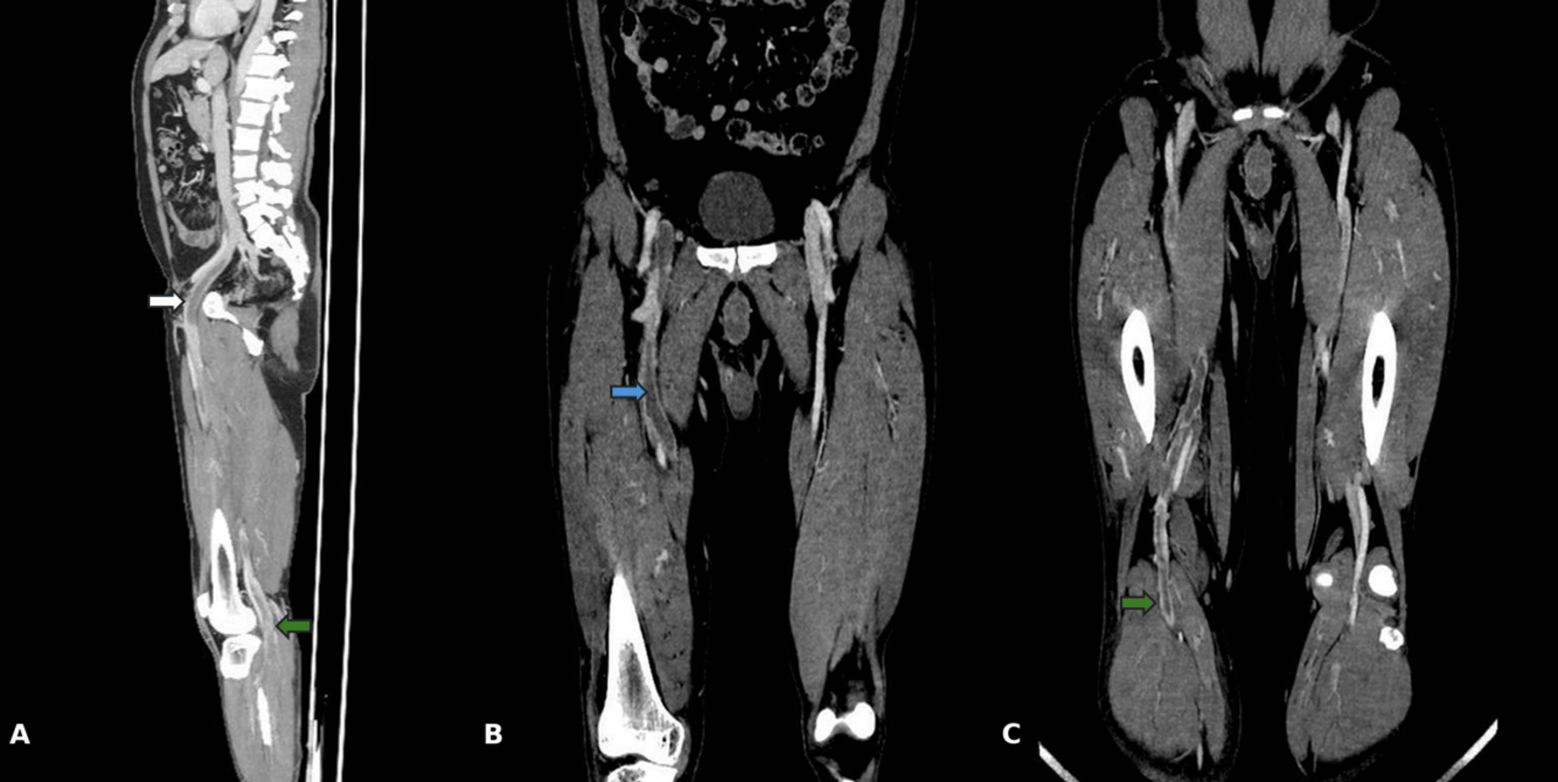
Contrast‑enhanced CT angiography demonstrating extensive right‑sided iliofemoral DVT with distal extension (A) Sagittal reconstruction shows a central low‑attenuation intraluminal filling defect in the right external iliac vein (white arrow) with distal propagation affecting the popliteal segment (green arrow).
